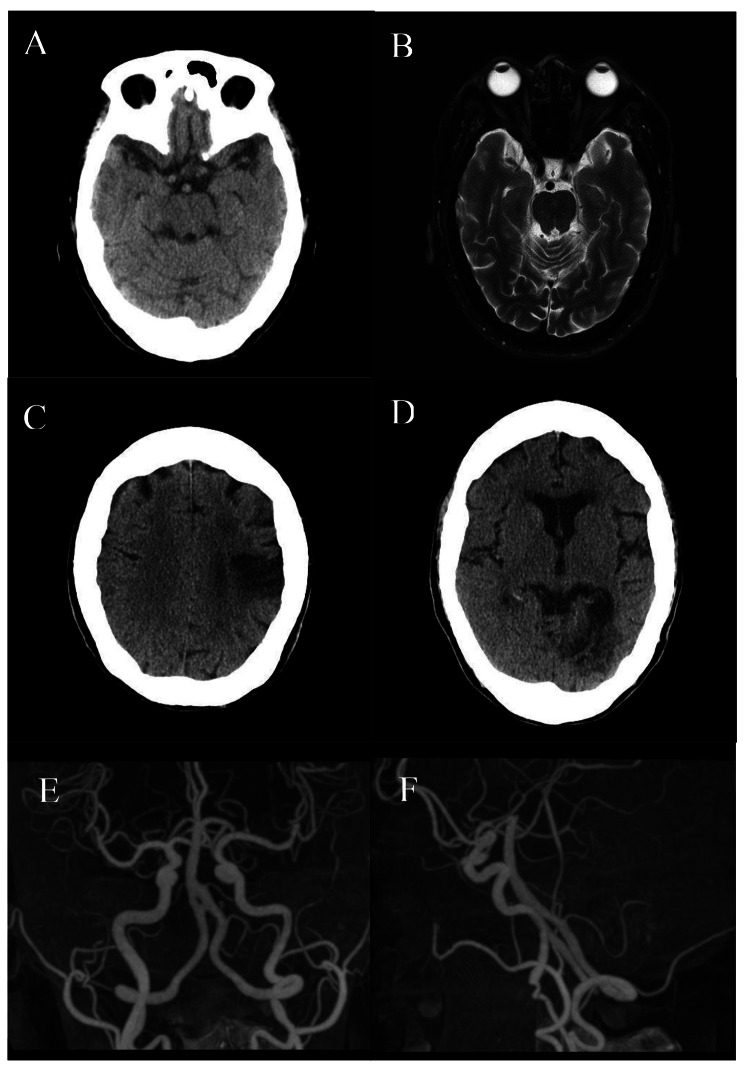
66-year-old FD female patient who suffered from recurrent ischaemic strokes. (A) Initial CT Brain showing prominent BA. (B) BA diameter measuring 5.3mm on axial T2-weighted MRI imaging. Recurrent ischaemic strokes (C) left parietal infarct in left middle cerebral artery territory (D) left occipital infarct in left posterior cerebral artery territory. Intracranial arterial dolichoectasia was appreciated on CT angiography in PA view (E) and lateral view (F). Both anterior and posterior vessels were elongated, tortuous and ecstatic
